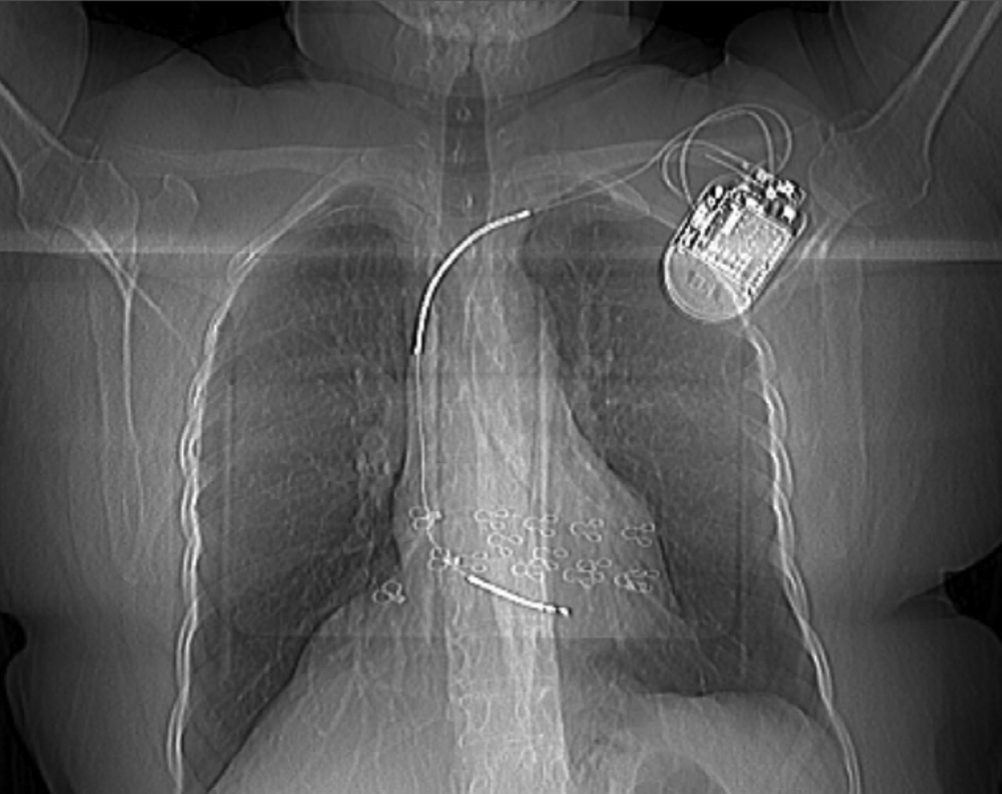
Scout image of Chest CT scan showing a single chamber ICD implant in the left upper chest wall.
